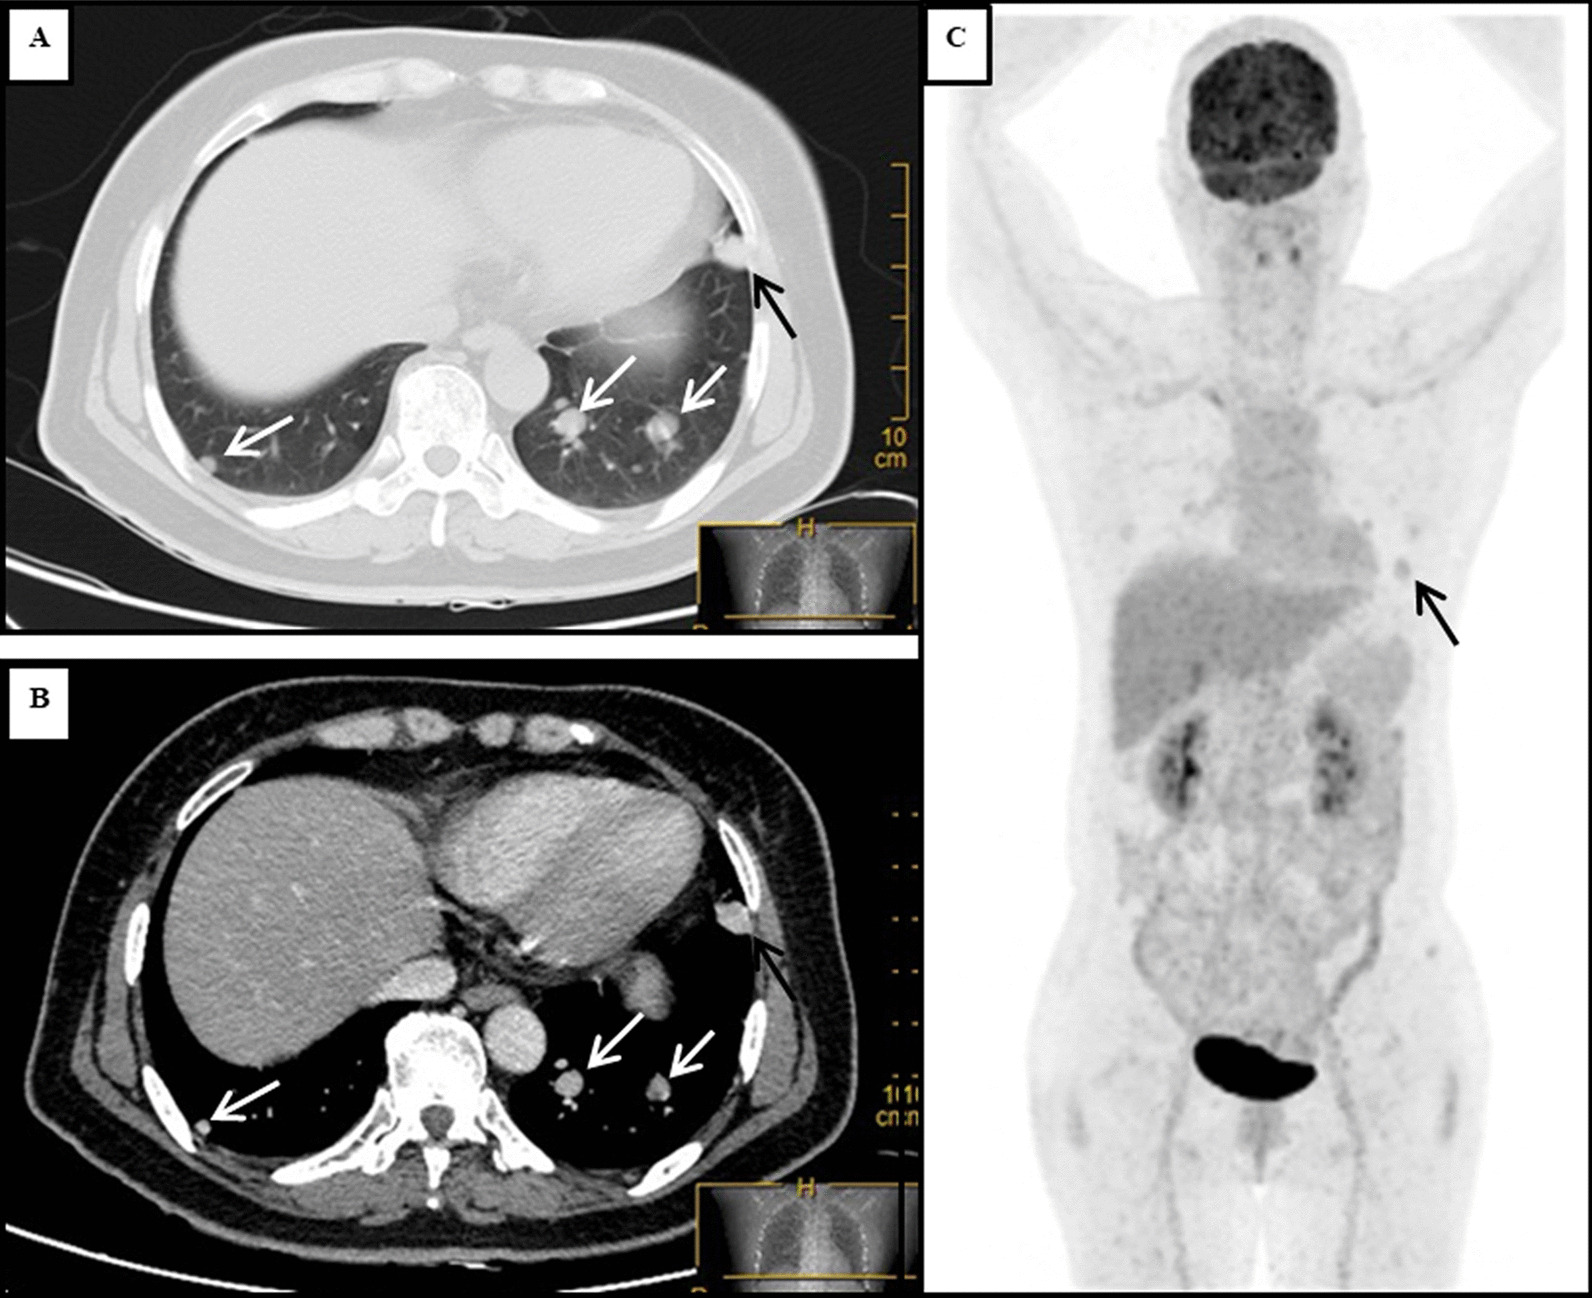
The chest CT scan and PET-CT examination showed multiple bilateral nodules in the patient’s lung lobes. The lung window (A) and longitudinal window (B) demonstrated that some round, well-defined and various-sized nodules were seen in both lungs (white arrow), the largest nodule was found in segment 5, and that nodule measured 15*14 mm (black arrow). (C) PET-CT indicated that there were multiple nodules in the bilateral lung lobes with mildly increased metabolism and that there was a larger nodule in the left lung (black arrow)
